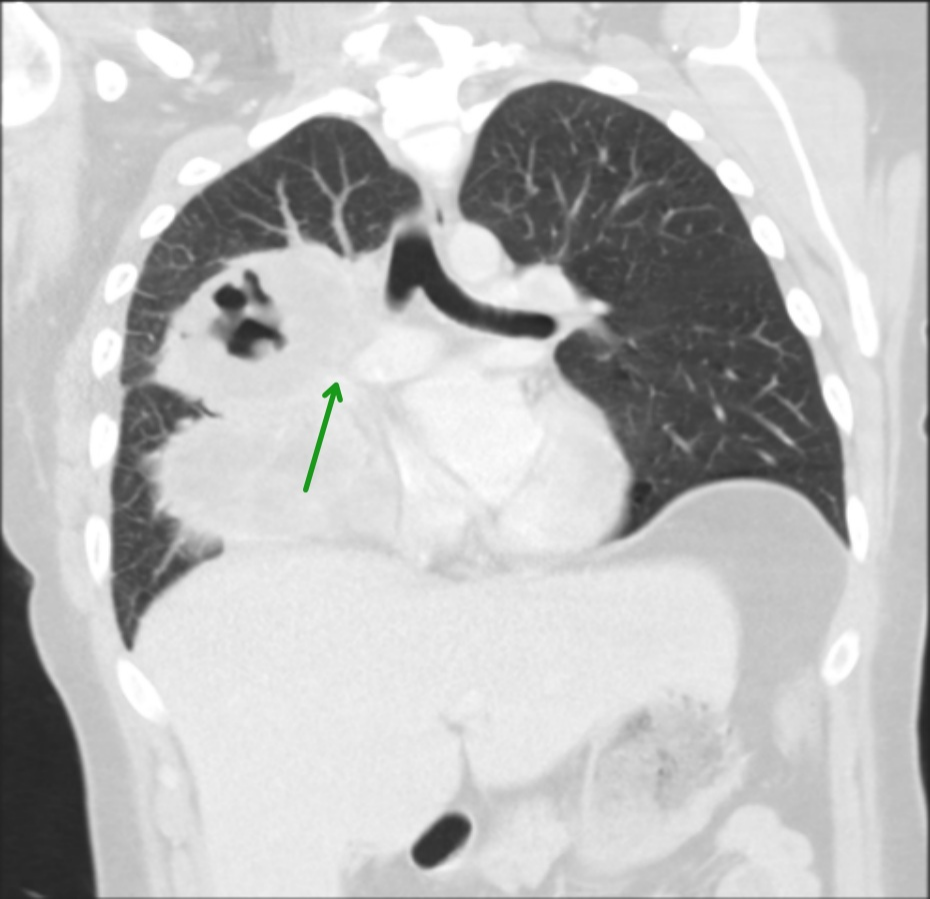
CT chest - coronal view Encasement of the right pulmonary artery (green arrow) is visible.
